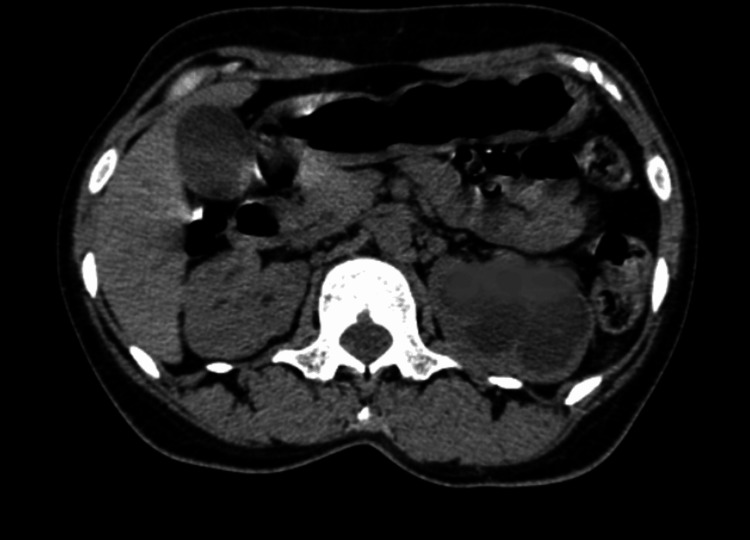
The CT scan shows complete resolution of right renal emphysematous pyelonephritis after oral antibiotics
